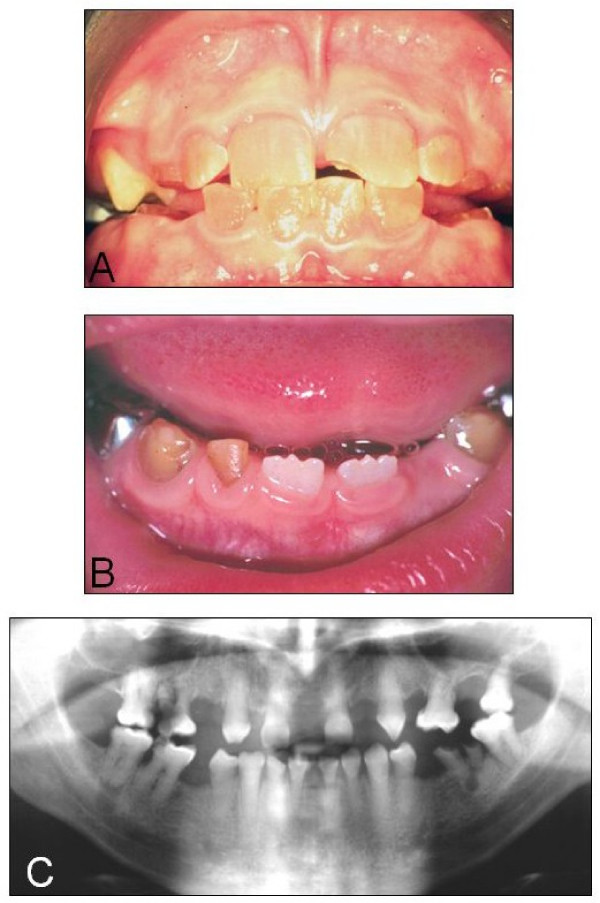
Clinical features of the inherited dentine disorders. A. Dentinogenesis imperfecta: The teeth are translucent and often roughened with severe amber discolouration. B. Dentine dysplasia: The primary teeth are translucent and amber in colour whereas the erupting secondary central incisors are of normal appearance. C. Dentine dysplasia: Radiograph showing the thistle-shaped pulp chambers of the secondary teeth.
